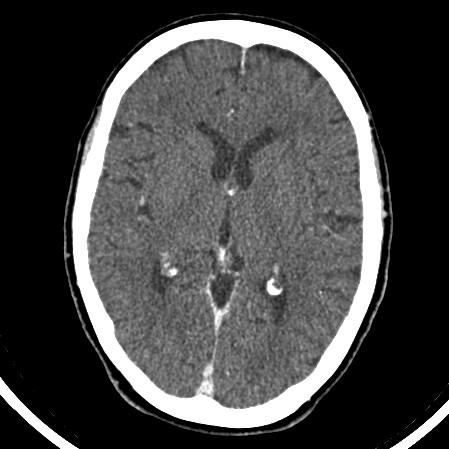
Computed tomography (CT) image of our patient's brain on presentation, without significant acute pathology.
